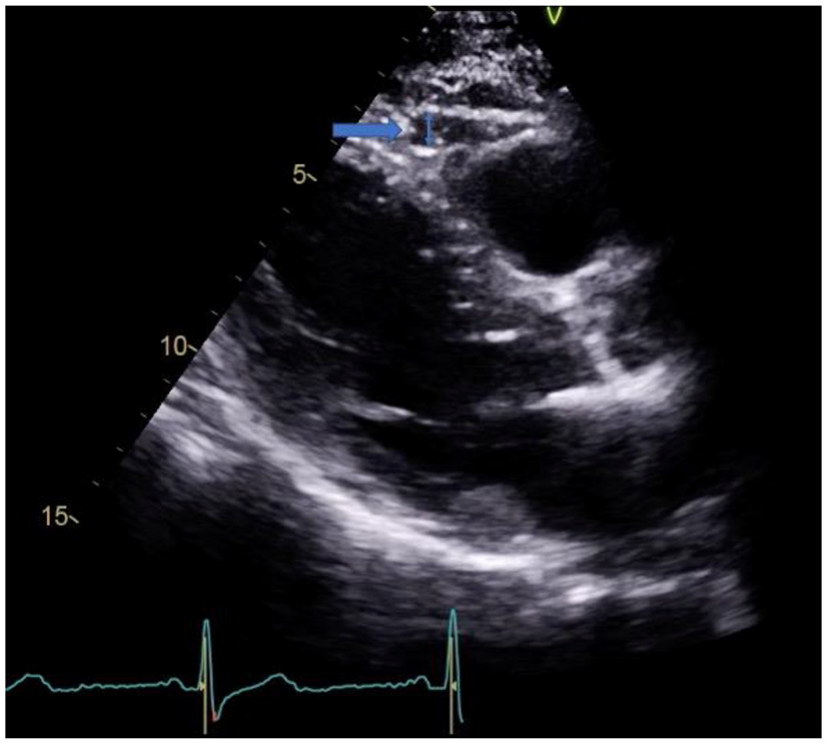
EAT in parasternal long axis view.
